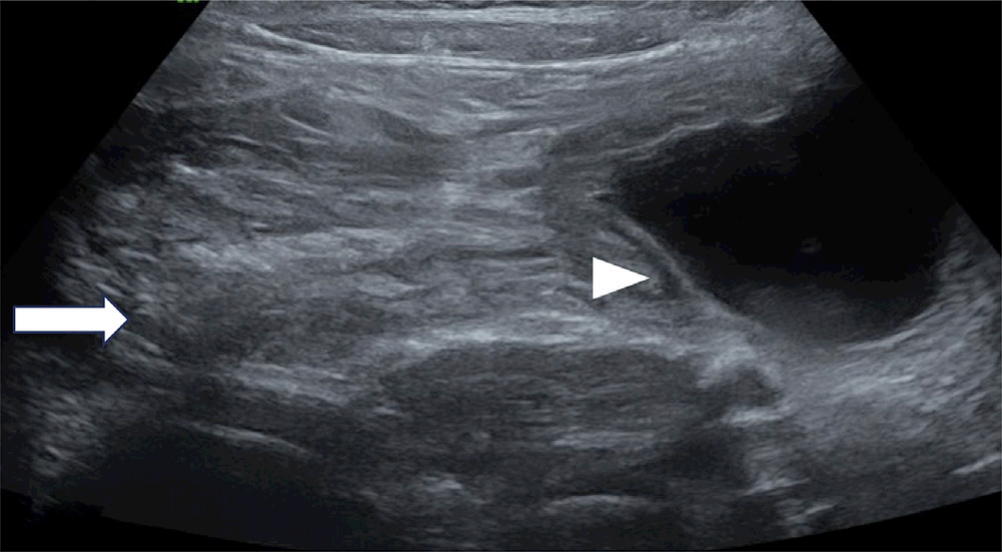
Longitudinal ultrasound image showing thickened bowel wall consistent with intussusception, demonstrating the characteristic “*pseudo-kidney*” appearance with alternating hypoechoic and hyperechoic layers (white arrow). Adjacent to this, a well-defined anechoic cyst with smooth walls is visible (white arrowhead), suggestive of a cecal duplication cyst.
